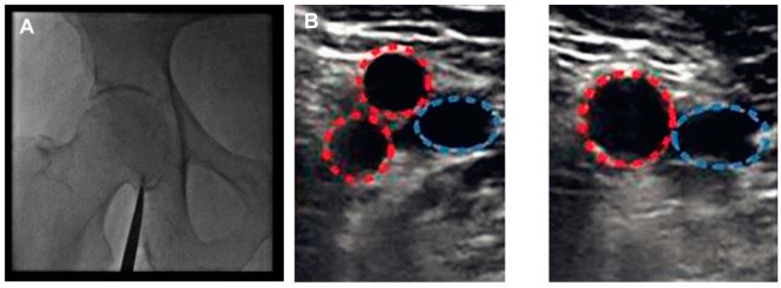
(A) Fluoroscopy is used in order to identify the femoral head, and then, the lower edge of the femoral head is marked with a hemostat. (B) Ultrasound is used in order to identify the femoral artery. When both superficial and profunda femoral arteries are visible, the probe has been placed under bifurcation (left panel), while when only the common femoral artery is visible, the probe is above femoral bifurcation (right panel), indicating the appropriate puncture site. Common, profunda and superficial femoral arteries are indicated with red color while the femoral vein is indicated with blue color. Reprinted from JACC Cardiovasc Interv. Volume 10, issue 22. Sandoval Y, Burke MN, Lobo AS, et al. Contemporary arterial access in the cardiac catheterization laboratory. Pages 2233–2241, 2017 [31]. Used with permission from Elsevier.
